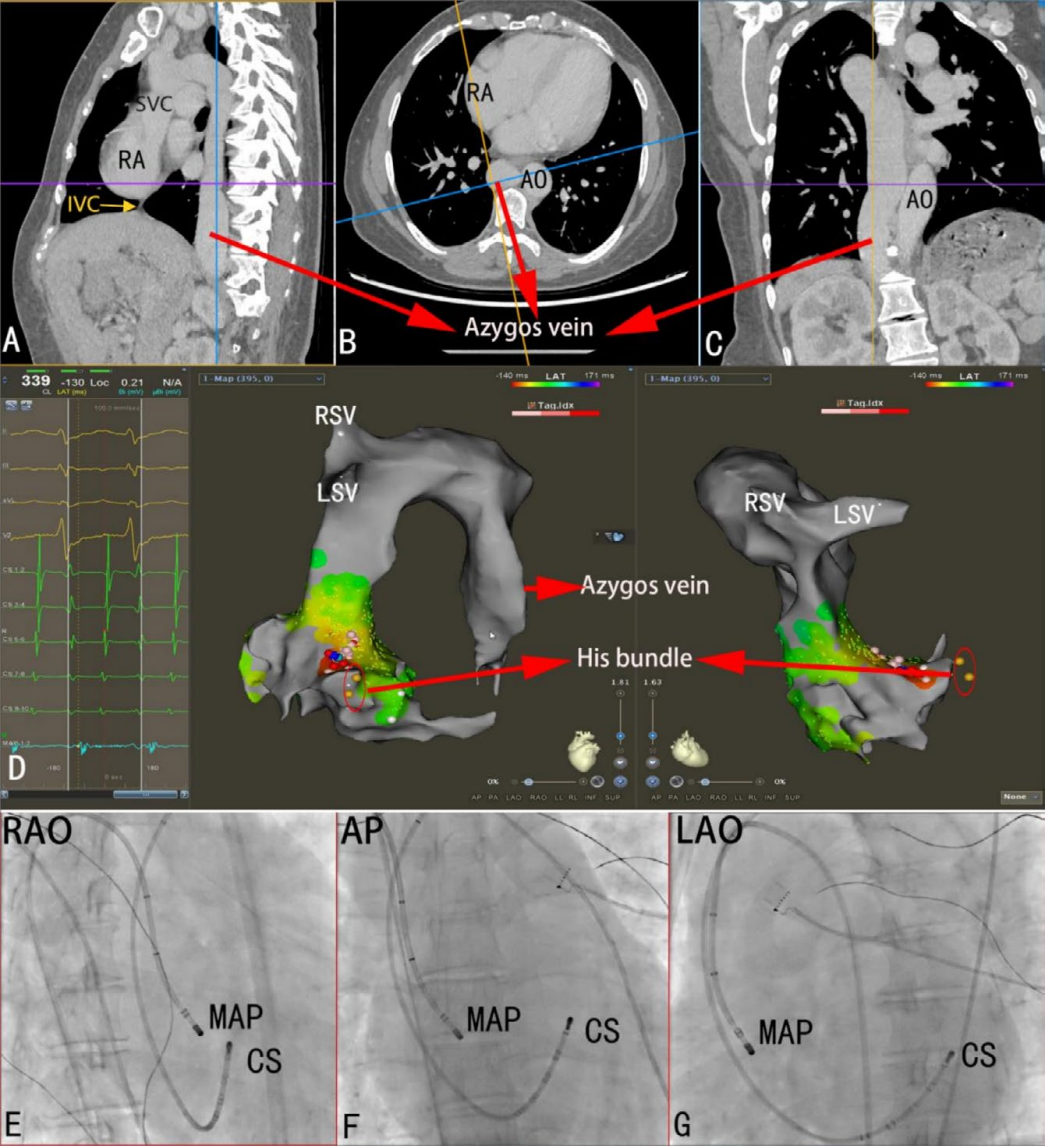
(A–C) Preoperative CTA demonstrating IVC occlusion (yellow arrow) and collateral drainage via azygos vein. (D) 3D electroanatomical map highlighting earliest activation site (blue dot) at anterior interatrial septum. (E–G) Fluoroscopic images confirming catheter positions (CS and mapping catheters) during ablation.
